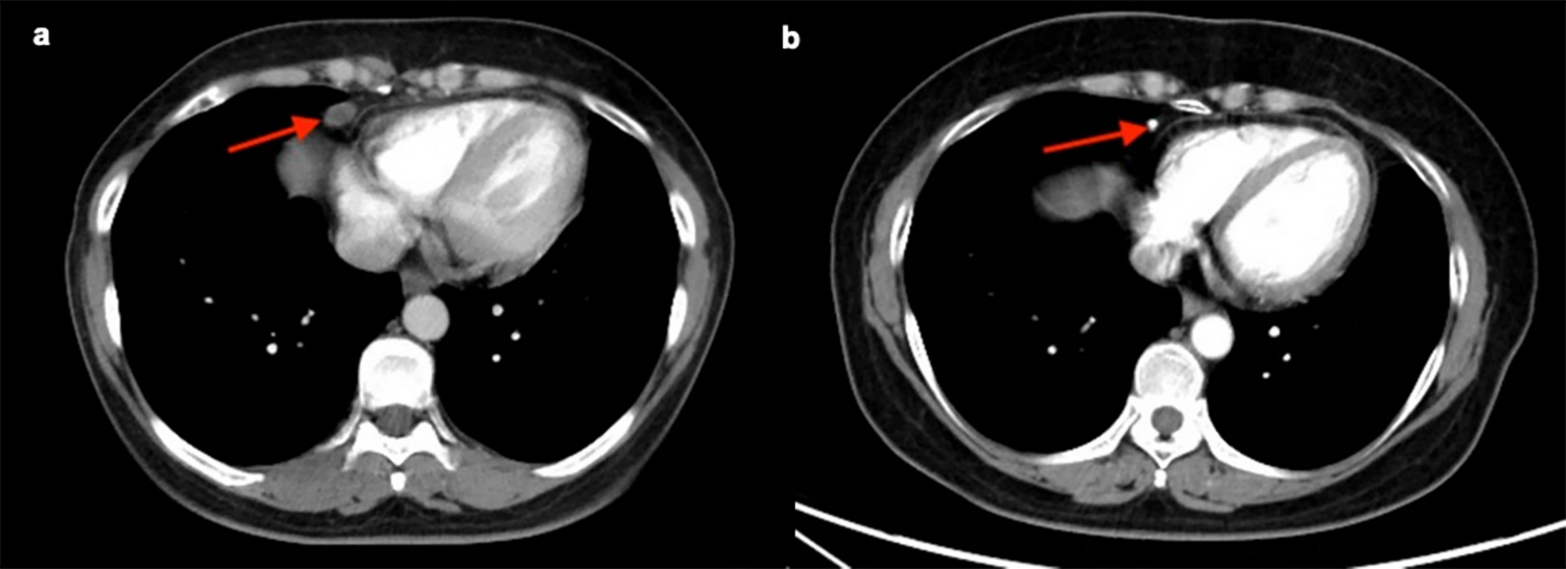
Staging CT image of a patient with FIGO stage IIIC ovarian cancer who presented with an enlarged cardiophrenic lymph node (CPLN) of 9.7 mm in the short axis (A). The CPLN had decreased with a short axis diameter of 3.0 mm 4 years later, and the patient had no recurrence (B).
